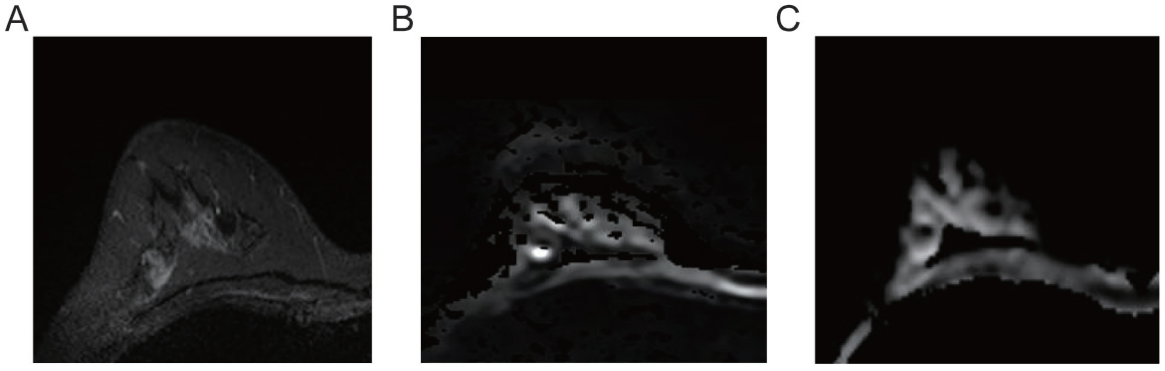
Right breast ductal carcinoma *in situ* (Luminal A subtype). The lesion appears as isointense on T2-weighted imaging (A). Diffusion-weighted imaging (DWI, b=800) shows high signal intensity (B), while ADC imaging demonstrates slightly increased signal intensity (C).
